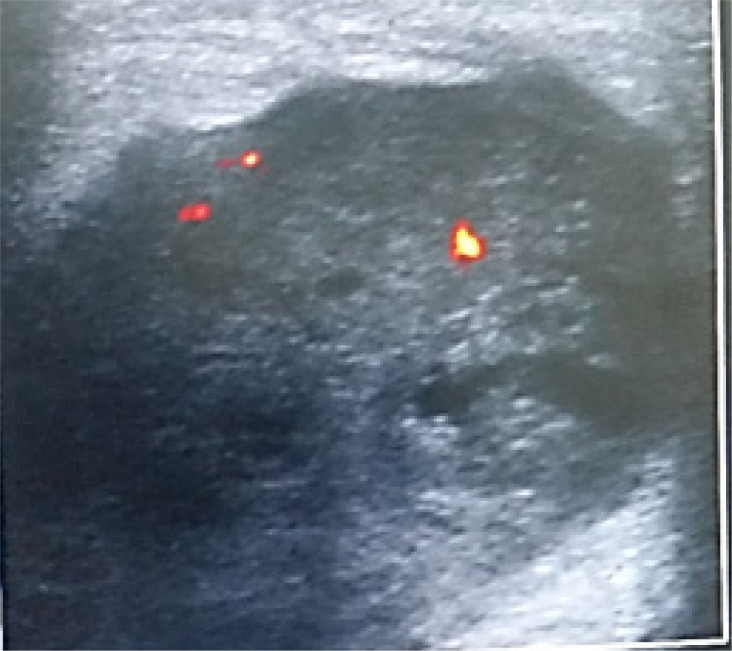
Complex cyst (type 4) > 50% solid with cystic foci A 55-year-old female with a hard non-tender left breast mass Ultrasound scan showing a predominantly solid mass which is vascular, with cystic spaces and angular margins Diagnosis: Invasive ductal carcinoma non-otherwise specified
